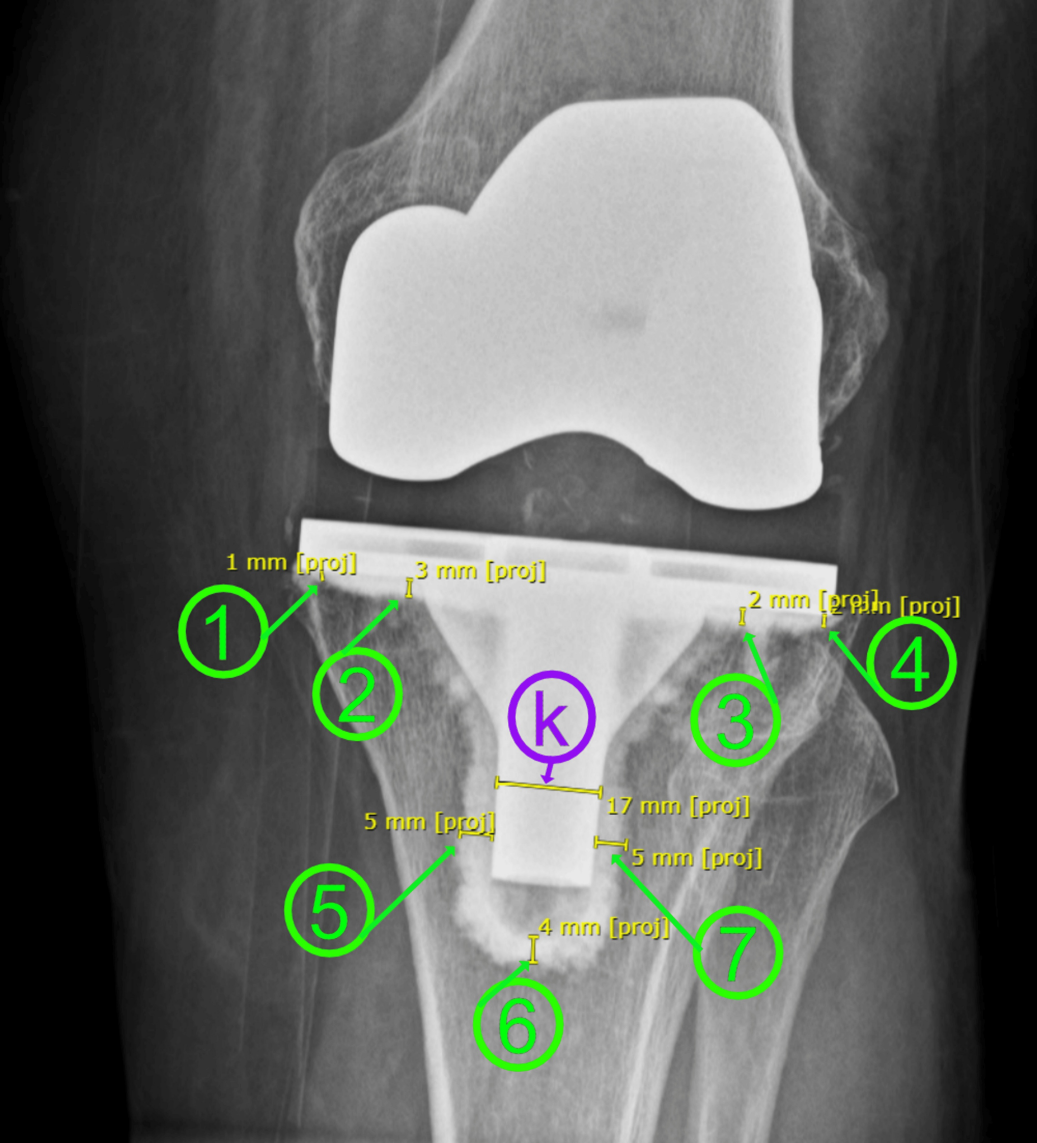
Measurement of cement penetrations on AP knee X-rays The green circles containing numbers 1-7 indicate the measured respective zones from zone 1 to zone 7. The purple circle containing the letter "k" indicates the keel diameter measurement. AP - anteroposterior Based on the Knee Society Roentgenographic Evaluation System [16].
